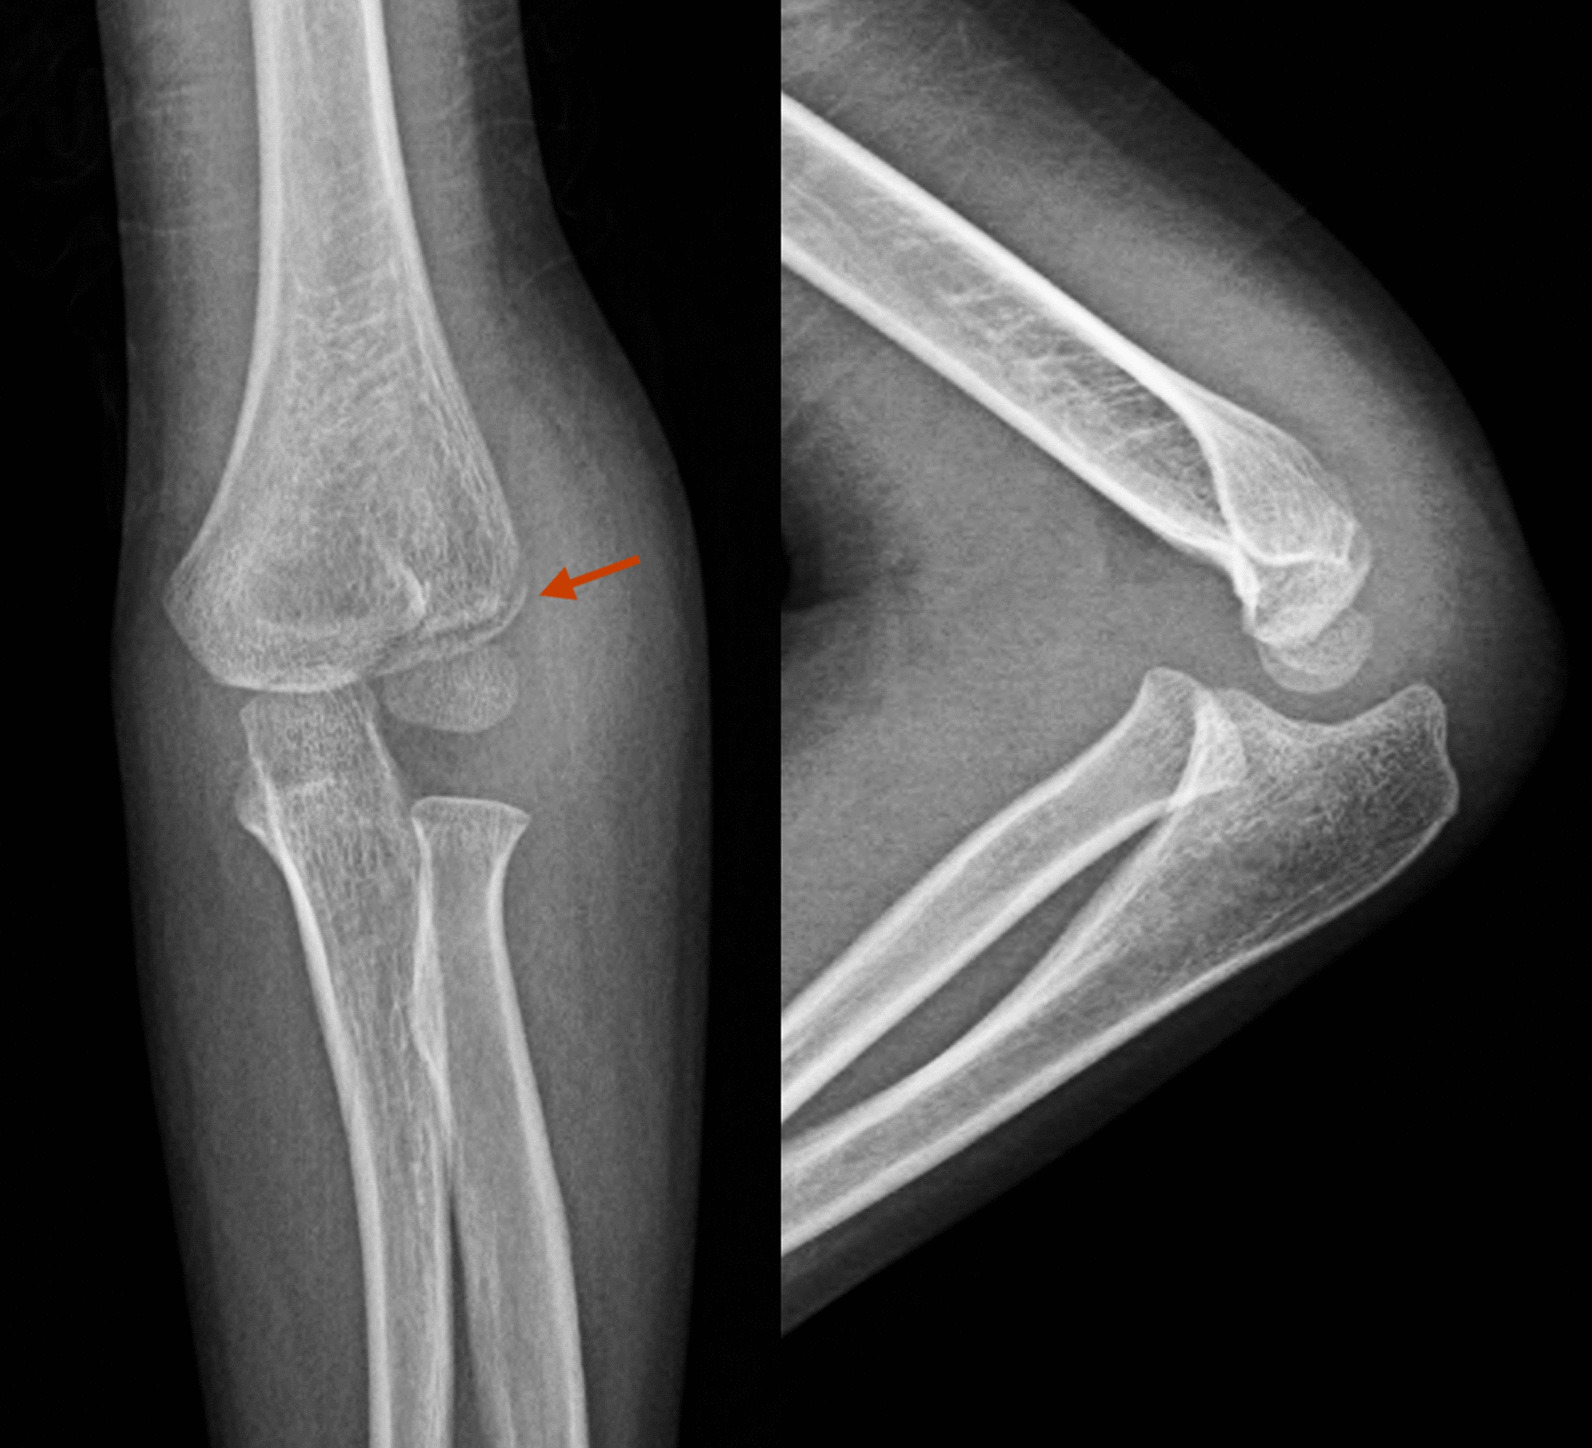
The frontal and lateral radiograph of Left elbow in a 6 years old boy with HLCF. It showed the distance of the lateral fracture space was about 1 mm in frontal image (The arrow showed the fracture line); there was no obvious lateral fracture in lateral image, but positive fat pad sign. HLCF, Humeral lateral condyle fracture
